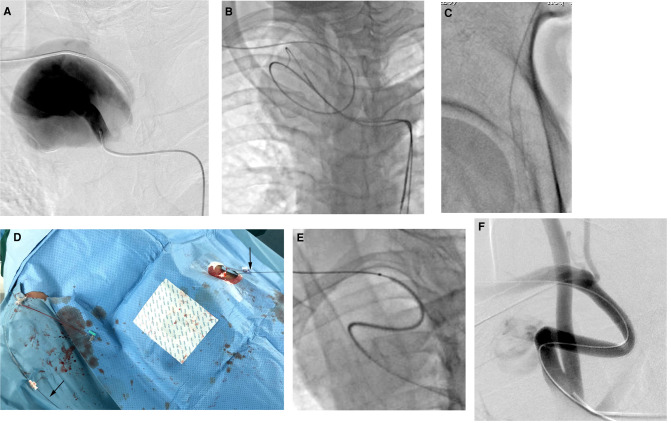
(A) Selective angiography *via* the left femoral artery verified the CTA findings. (B) The guidewire was circled along the shape of the aneurysm instead of along the shortest path. (C) The tip of the guide wire was introduced into a sheath placed in the femoral artery. (D) The guidewire was held out of the body, thus creating a through-and-through guide wire (black arrows). (E) The stent graft smoothly advanced to the intended position, 20 mm away from the orifice of the right subclavian artery. (F) Final angiography showed that the SAA was essentially excluded, although a slight endoleak was also observed.
